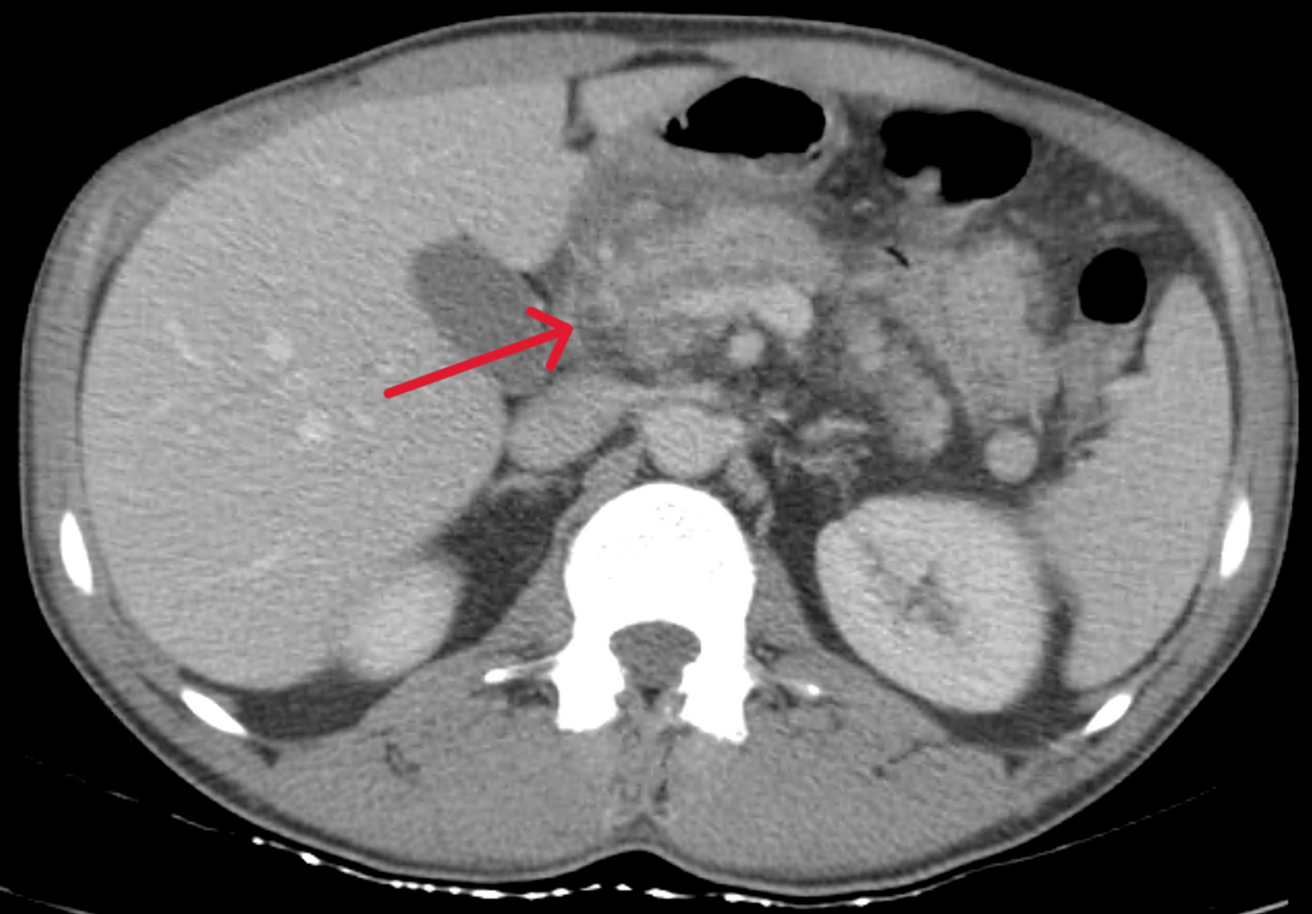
Abdominal CT scan The red arrow signals the diffuse swelling of the pancreas head CT: Computed tomography
